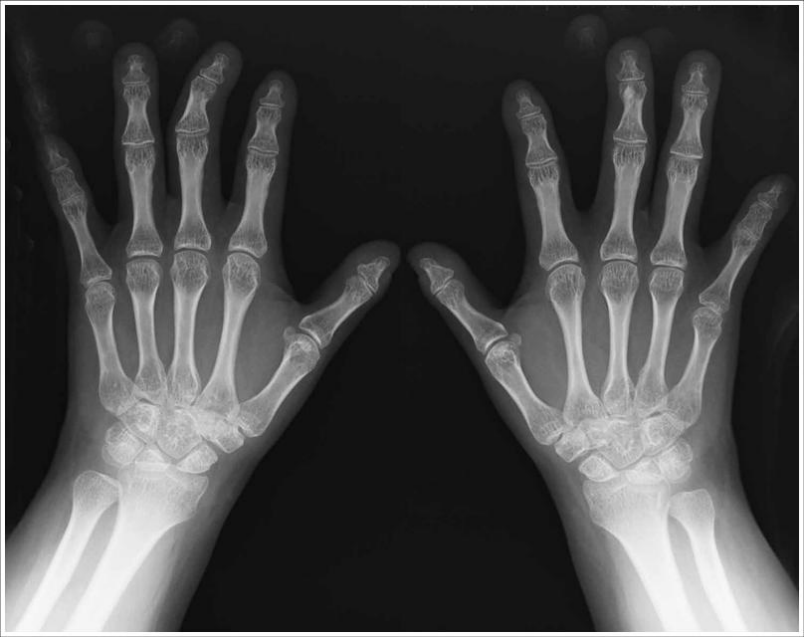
Radiographs of hands. Note mild shortening of the fifth metacarpal of the hands.
